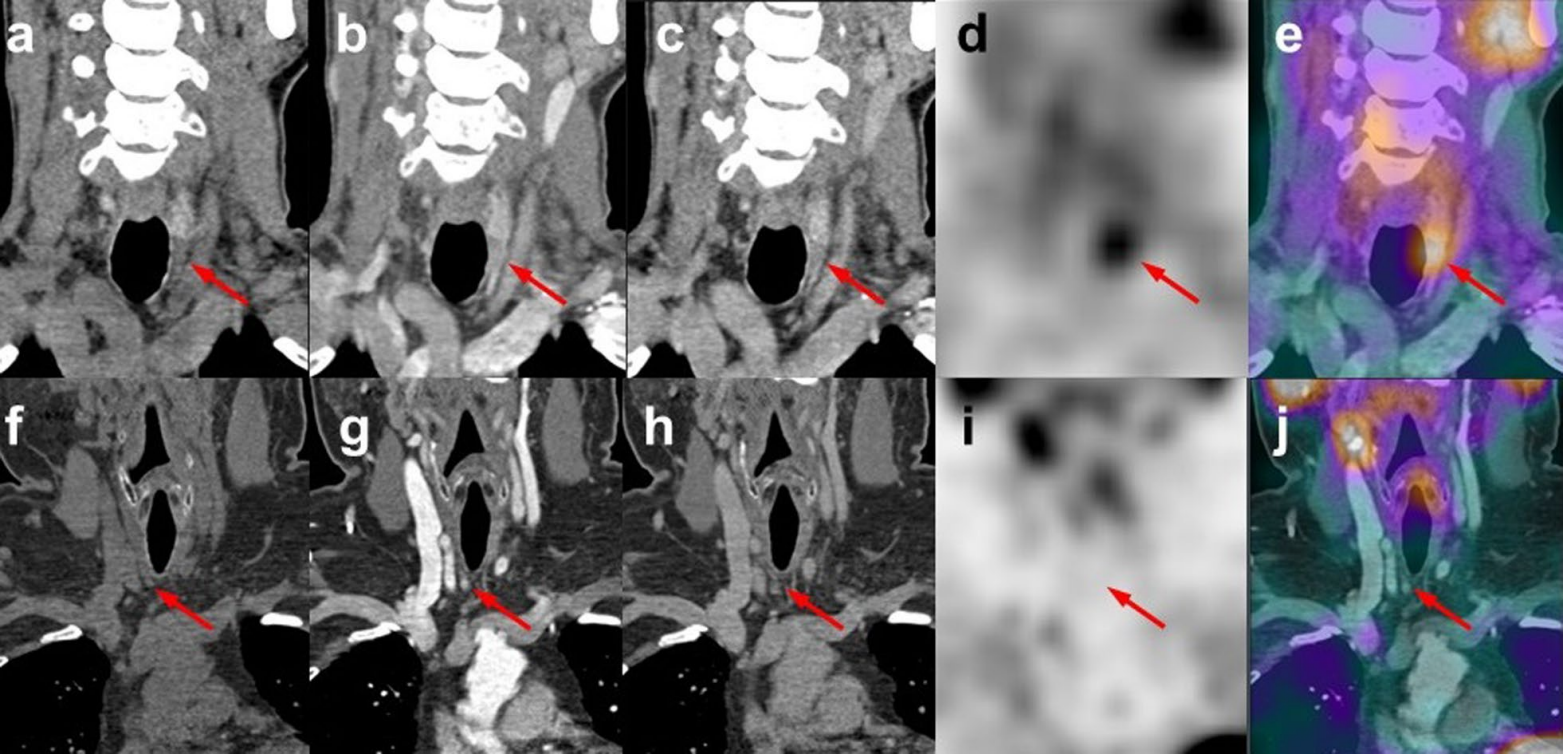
Multiphase SPECT/CT coronal views for 2 patients, upper and lower rows, respectively. Native phase (a and f), arterial phase (b and g), venous phase (c and h) and 90 min Tc-99m-Sestamibi SPECT (d and i) and fused SPECT and arterial CT phase (e and j). Arrows point to the anatomical localisation of the parathyroid adenomas with a weight of 222 mg and 205 mg for the upper and the lower rows, respectively
